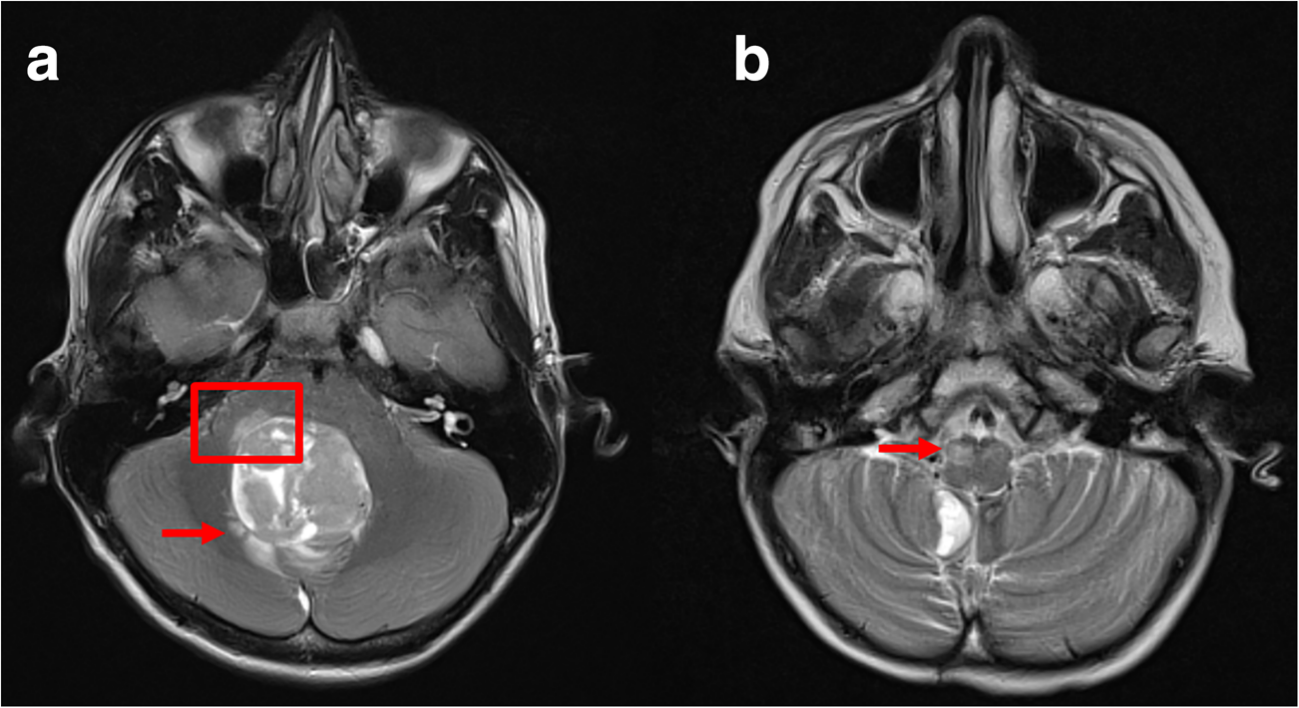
T2-weighted magnetic resonance imaging in the axial plane. Panel a shows preoperative imaging of a medulloblastoma with perifocal edema extending into the right middle cerebellar peduncle (red box) and right dentate nucleus (red arrow). Panel b demonstrates right-sided hypertrophic olivary degeneration (red arrow) occurring 5 months after medulloblastoma resection as a morphological correlate of postoperative cerebellar mutism
